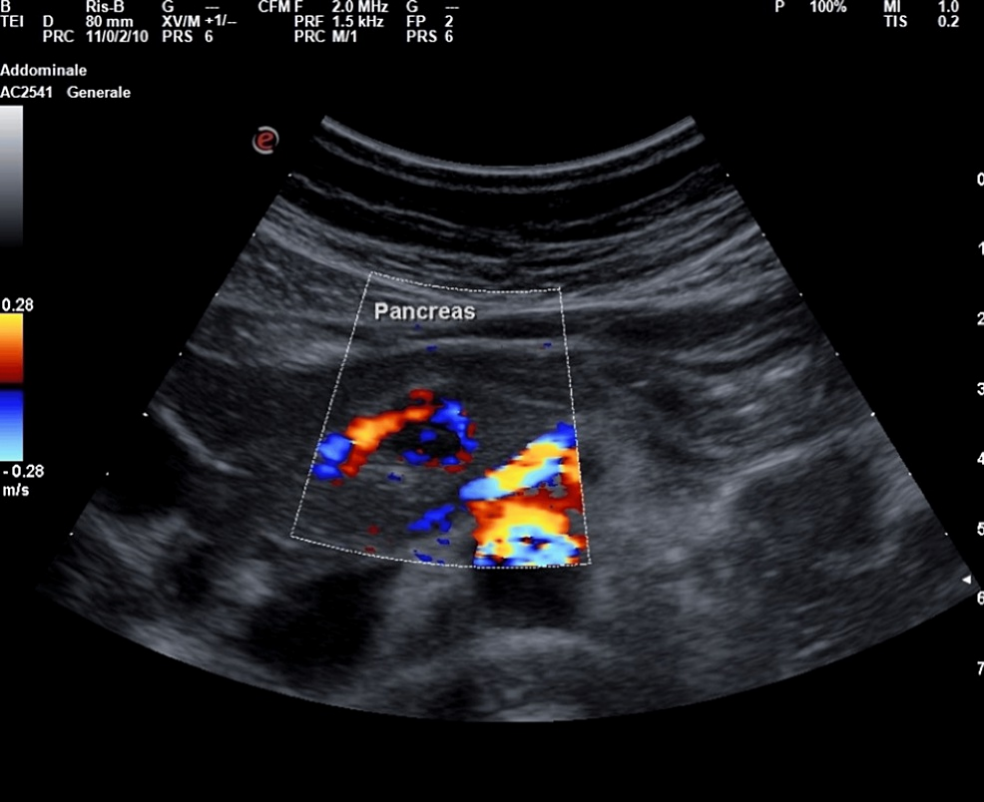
Bedside US of our patient conducted with Esaote MyLab Omega model with abdominal probe and preset; the lesion showed intense peripheral vascularization at the color signal taking the shape of a short rod. US: ultrasonography
